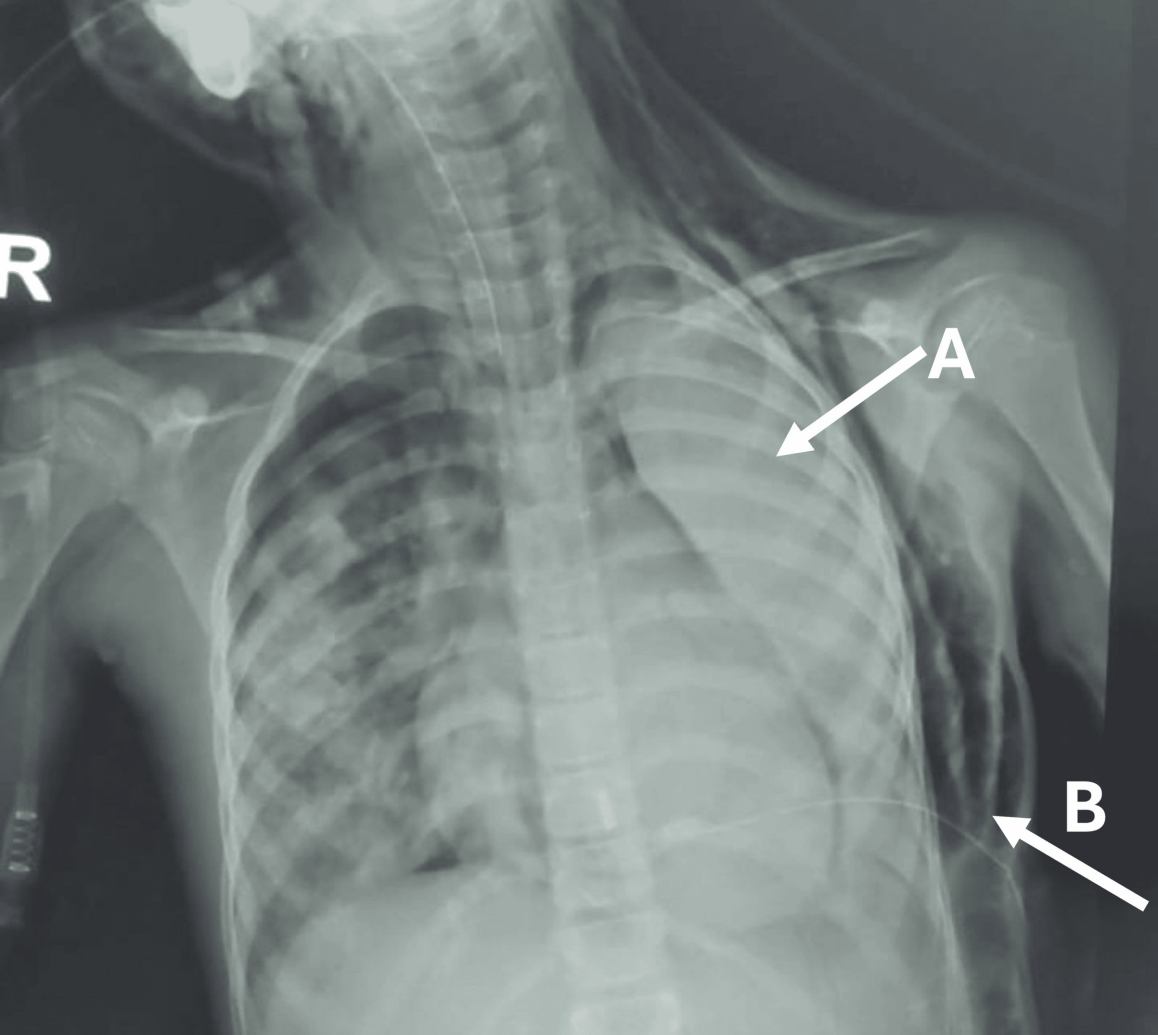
Chest radiograph during severe acute chest syndrome. Chest radiograph obtained during clinical deterioration showing near-complete opacification of the left hemithorax (arrow A) consistent with complete left lung collapse, with associated left-sided pleural pathology and subcutaneous emphysema of the left chest wall (arrow B) in the setting of severe acute chest syndrome.
